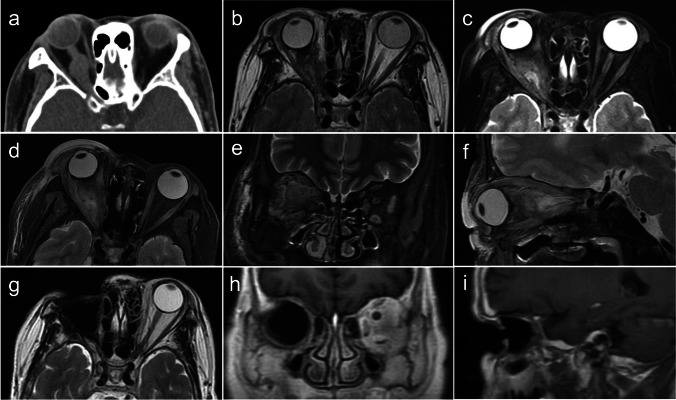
Case 2 presentation. Computed tomographic (CT) and MRI showing a right-sided recurrent ONSM underwent lateral orbitotomy approach (LOA) in April 2014. After LOA, there was no sign of recurrence. a CT image in October 2012; b axial T2-weighted MR image in January 2013; c axial T2-weighted MR image with Gd enhancement in February 2014; d–f axial, coronal, and sagittal T2-weighted MR images in April 2014; g–i at 94-month postoperative axial, coronal, and sagittal T1/T2-weighted MR images
